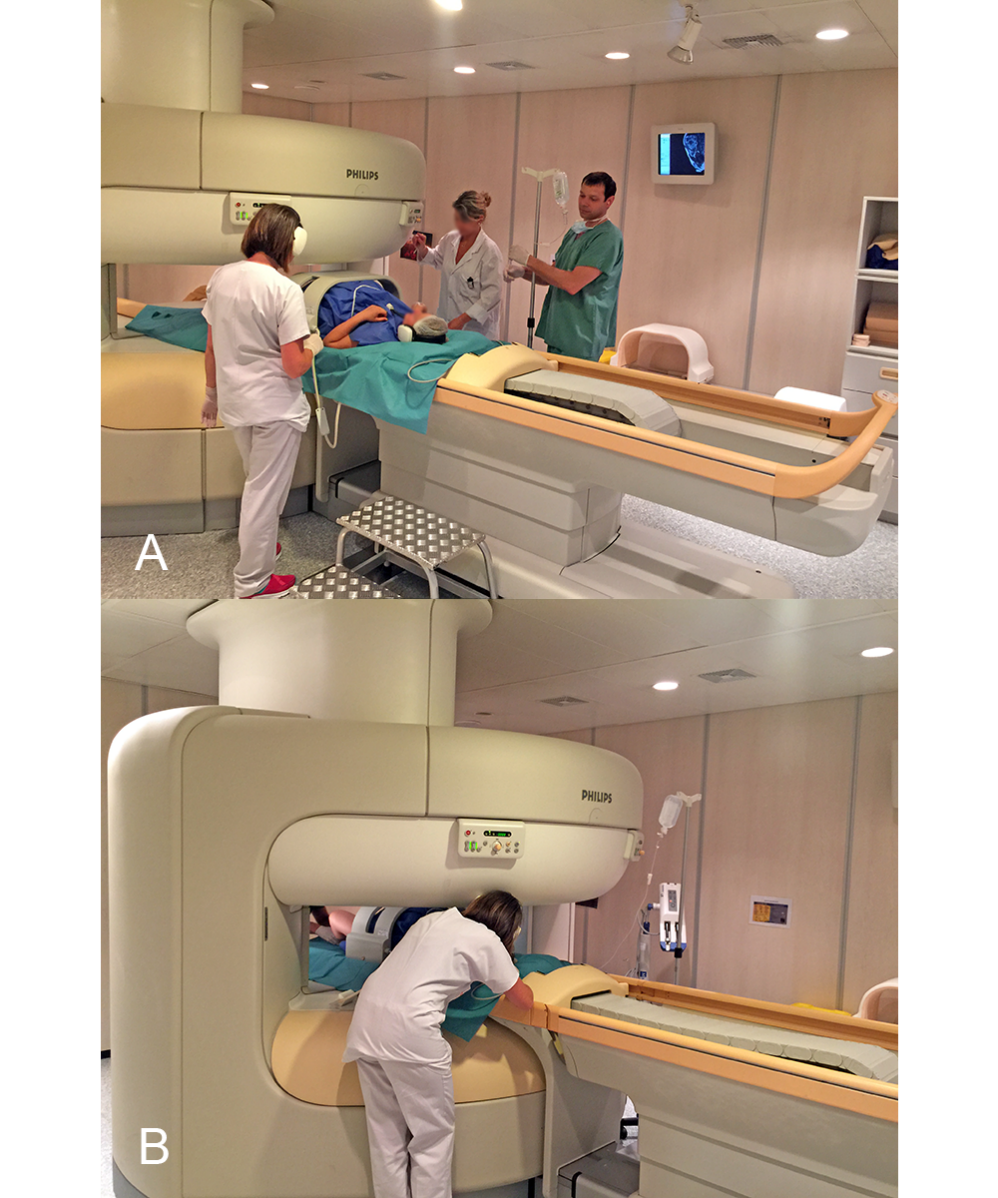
(A) Open magnetic resonance imaging (MRI) room with sterile drapes and a delivering patient. (B) Patient in the open-field MRI after antenna installation and being positioned inside the magnetic field.
